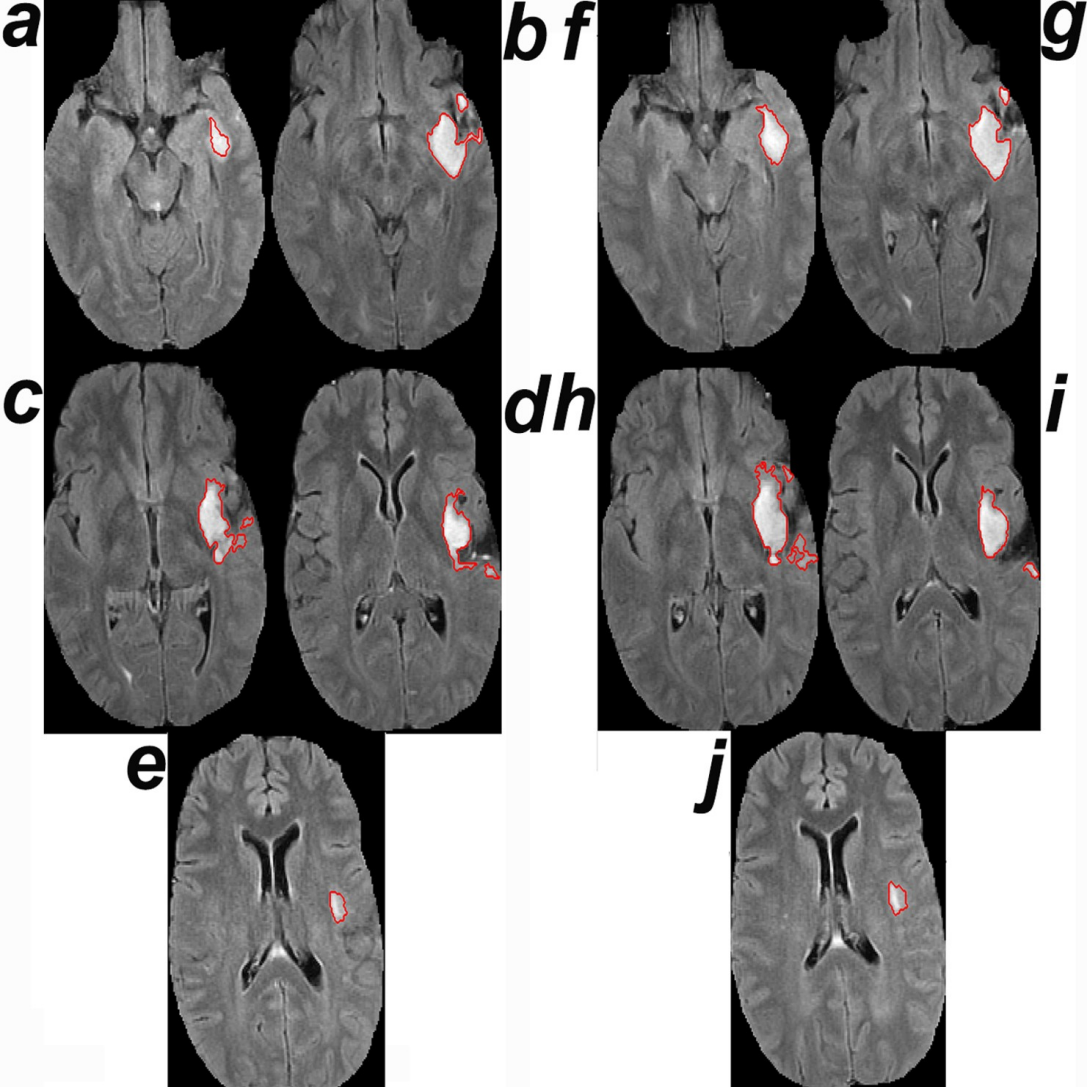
Growth away from the largest tumor section. (a–e) MRI of tumor 4385 (see S1 and S2 Datas) at time 0. (f–j) MRI at the time point of growth detection by CAD. The surface areas of the tumor segments in (a–j) are 268, 1,174, 1,240, 962, 246, 718, 1,262, 1,764, 994, and 282 pixels^2^, respectively. The tumor exhibits larger percent growth from baseline in the third dimension (compare a and f: 268 and 718) away from the section containing the largest tumor component at baseline (compare c and h: 1,240 and 1,764). The second MRI was deemed stable by visual comparison.
